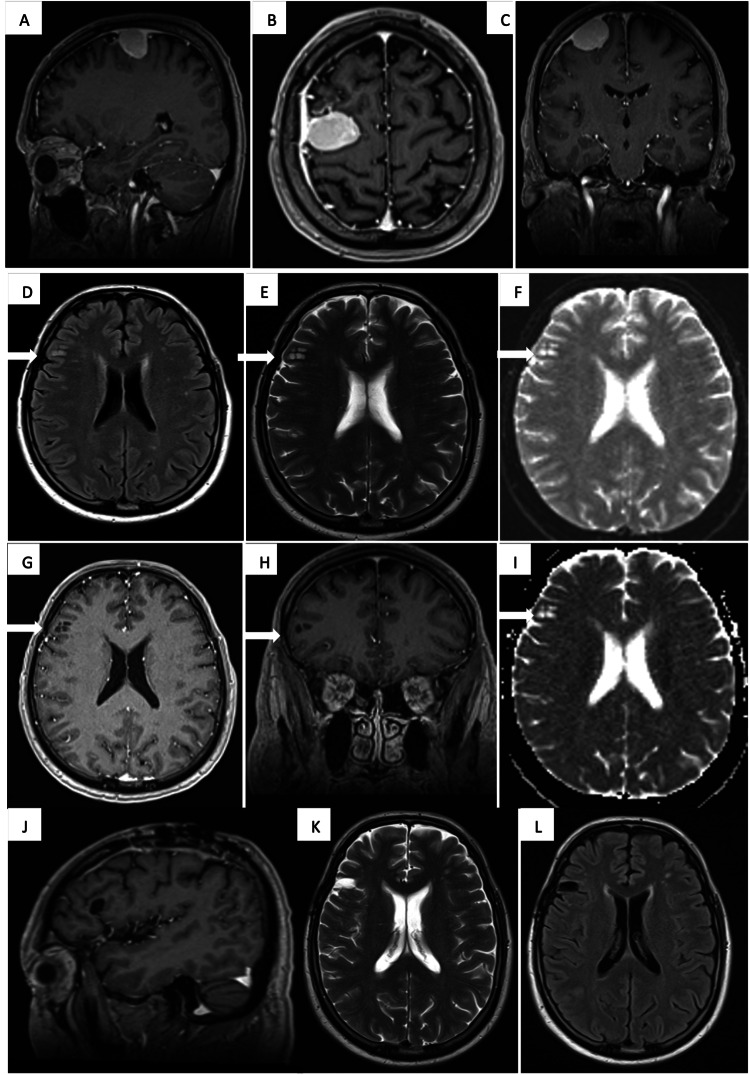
Magnetic resonance imaging Panels A, B, and C demonstrate right frontal meningioma. Panels D and E represent the FLAIR and T2 sequence where the white arrows point to the lesion diagnosed as MZBCL of MALT type while panels F and I are the diffusion-weighted image and apparent diffusion coefficient. Axial and coronal T1 with gadolinium are shown in panels G and H, respectively. Panels J, K, and L show a follow-up MRI obtained nine months after surgery, which confirms there is no residual or recurrent lesion. FLAIR: fluid-attenuated inversion recovery; MZBCL: marginal zone B-cell lymphoma; MALT: mucosa-associated lymphoid tissue
